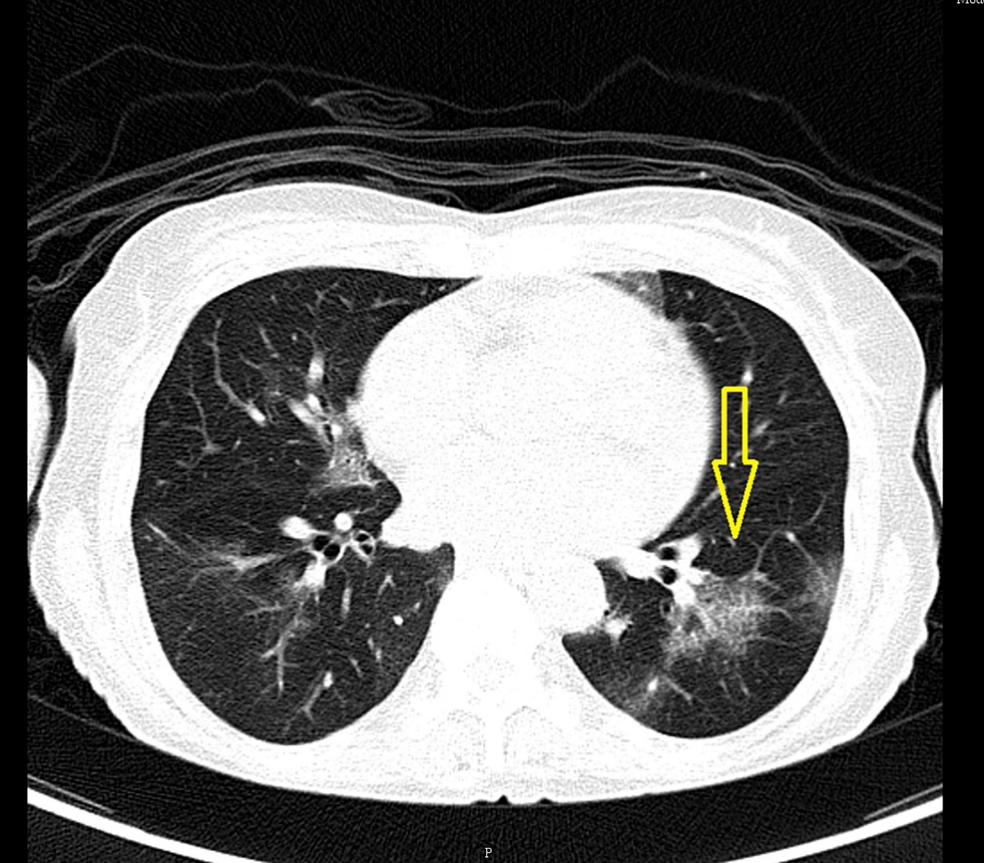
CT showing patchy ground glass opacity with interstitial thickening and subtle bronchiectasis changes in the left lower lobe and right middle lobe CT: computed tomography
